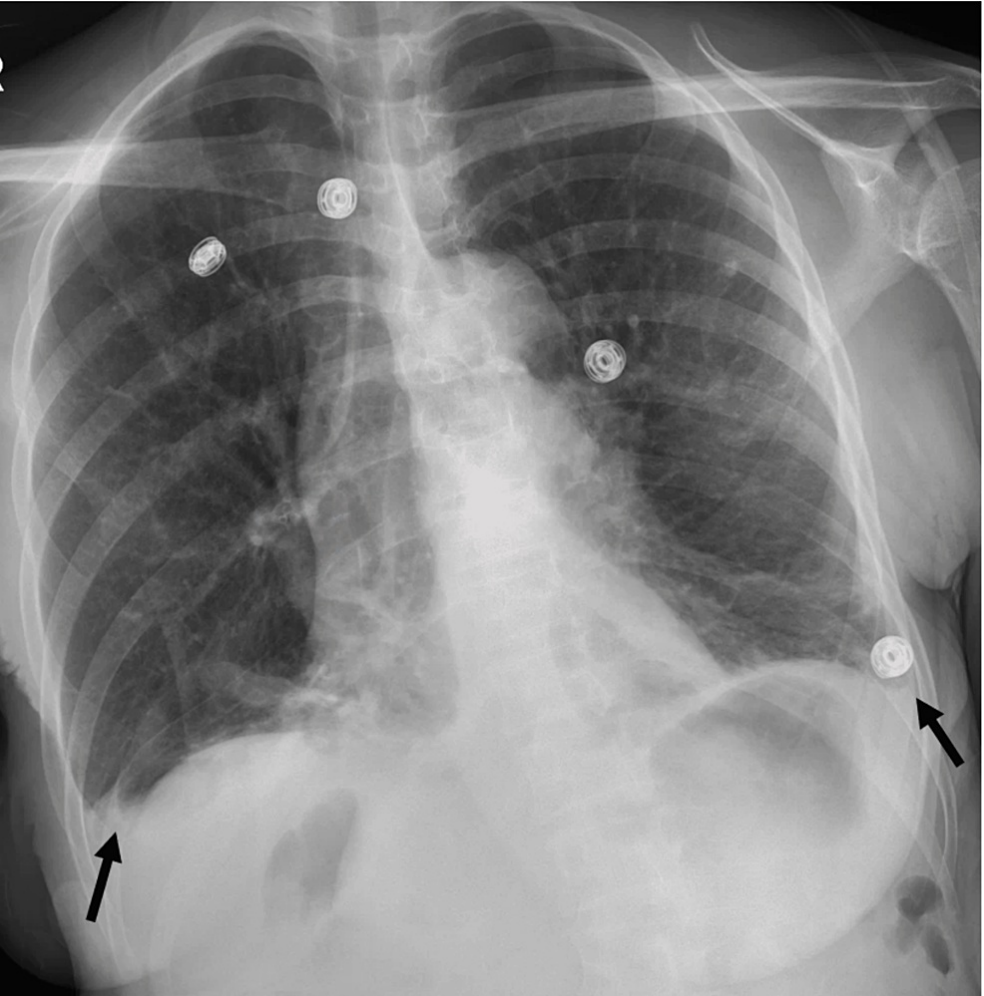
Chest X-Ray showing new small bilateral pleural effusions
